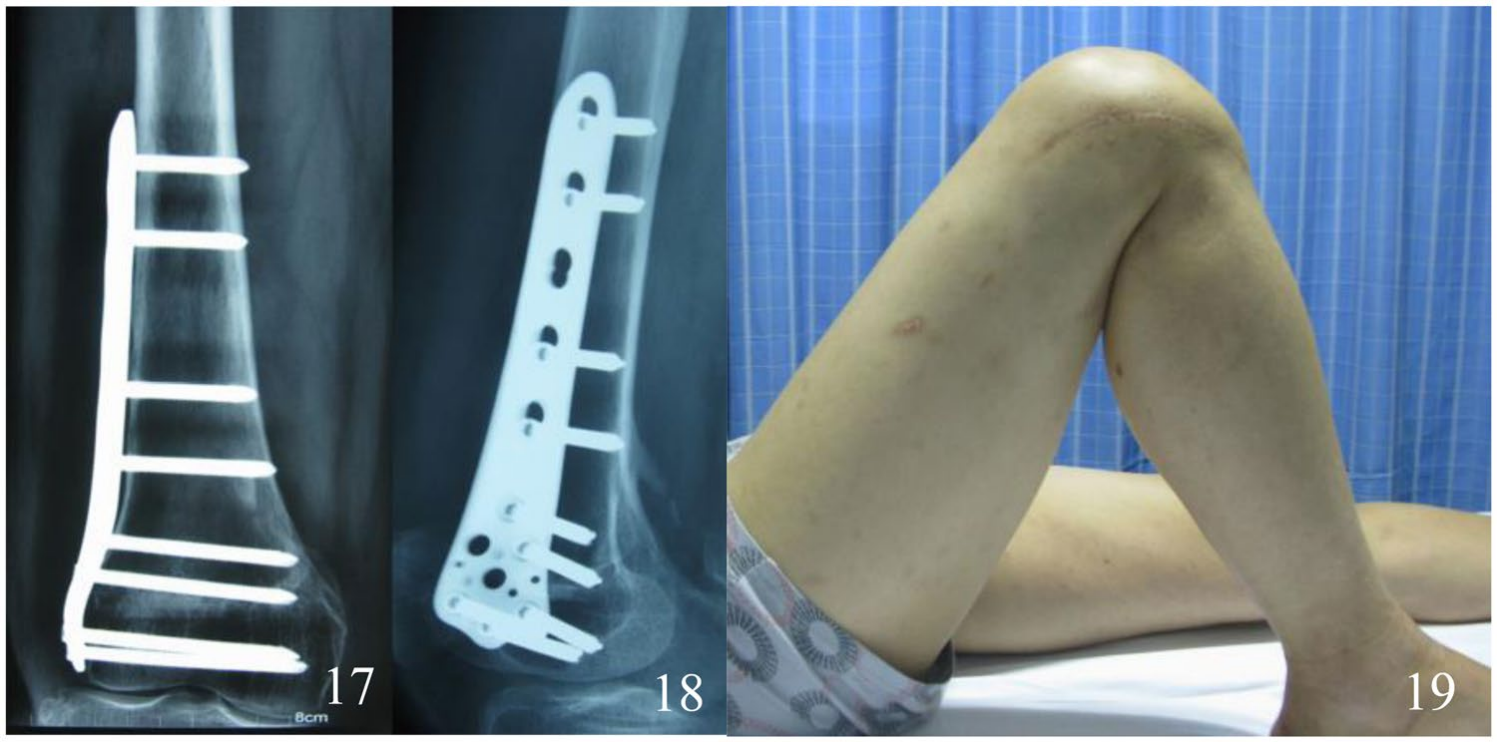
17, 18 the X-ray of femur, taken 18 months after operation, 19 the knee function of the patient, taken one year after operation.
